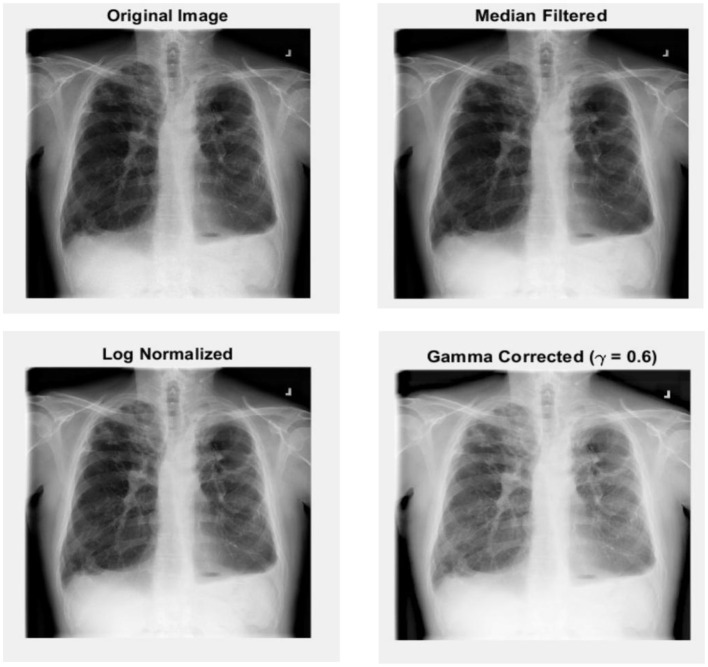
Representative preprocessing stages applied to chest X-ray images.
